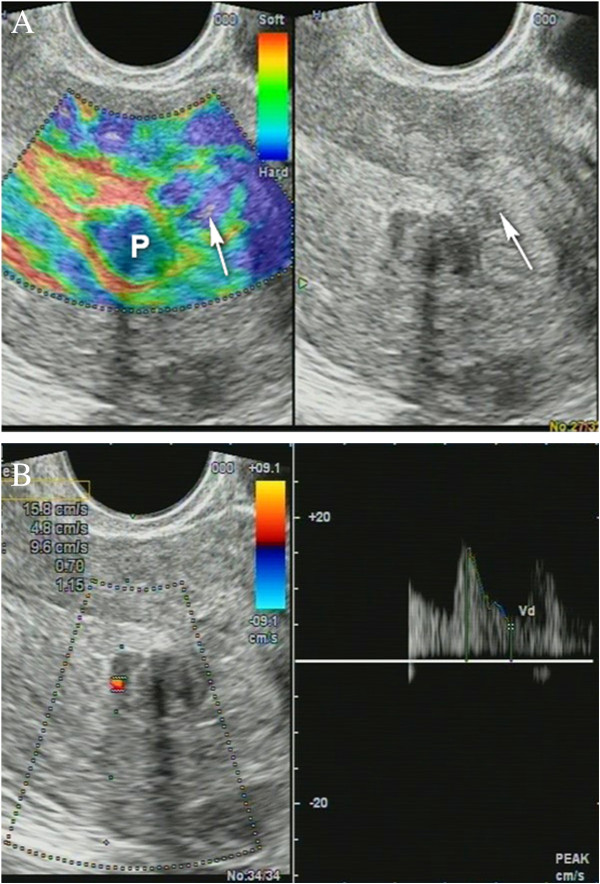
Endometrial polyp (*P*). (A) Stiff polyps on SEG, *arrow* indicates invisible polyp in *grey scale* that was detected on SEG; (B) Dopplerography of polyp vessel, RI = 0.7.
